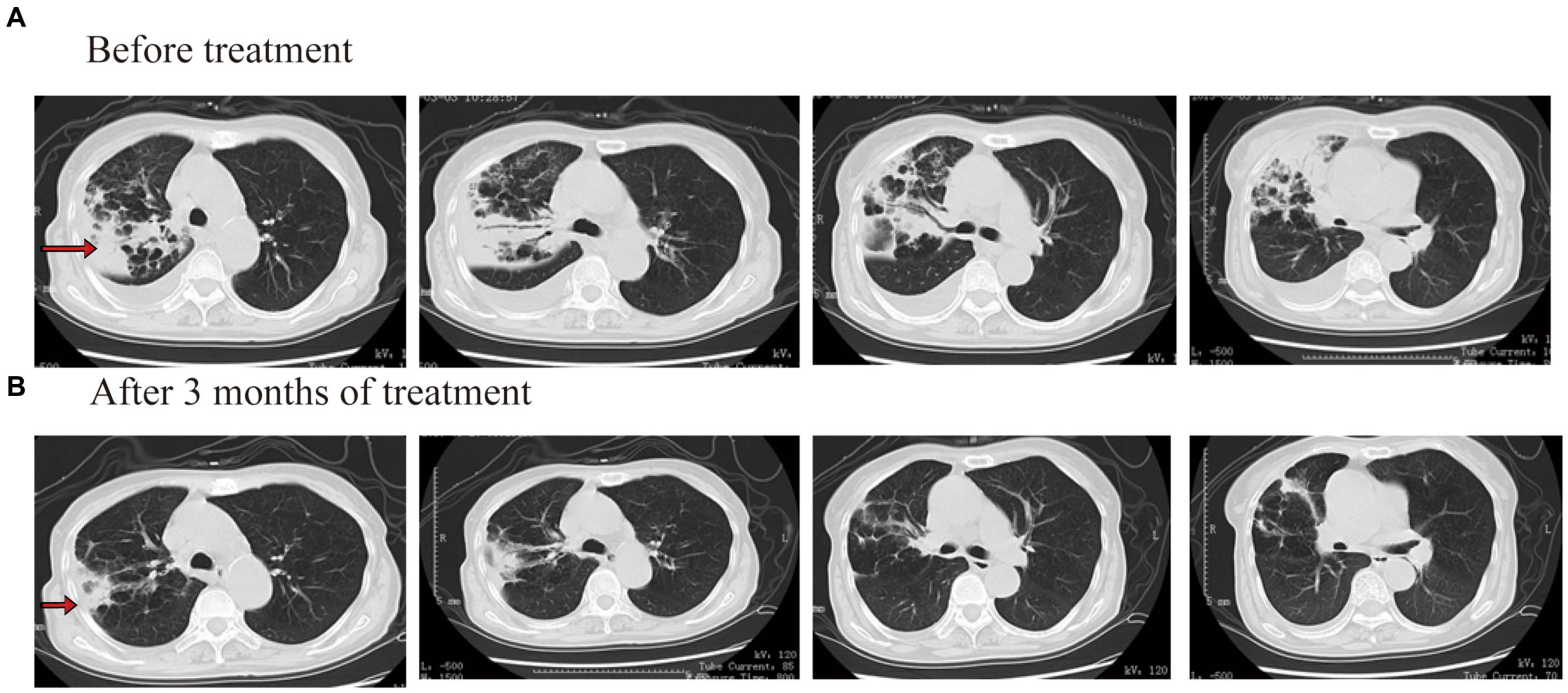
Chest CT scan images before and after treatment for case 1.
